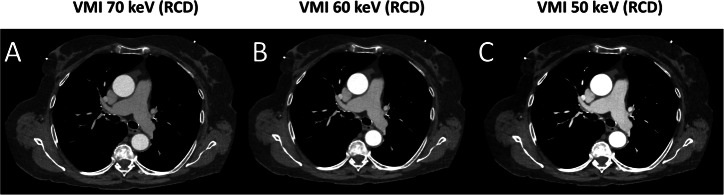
Representative axial images (window width/window level: 700/80 HU) of the same patient acquired with reduced contrast media dose (RCD), combined with virtual monoenergetic images (VMI) at 70 keV (A), 60 keV (B), and 50 keV (C) on a photon-counting detector CT (PCDCT)
